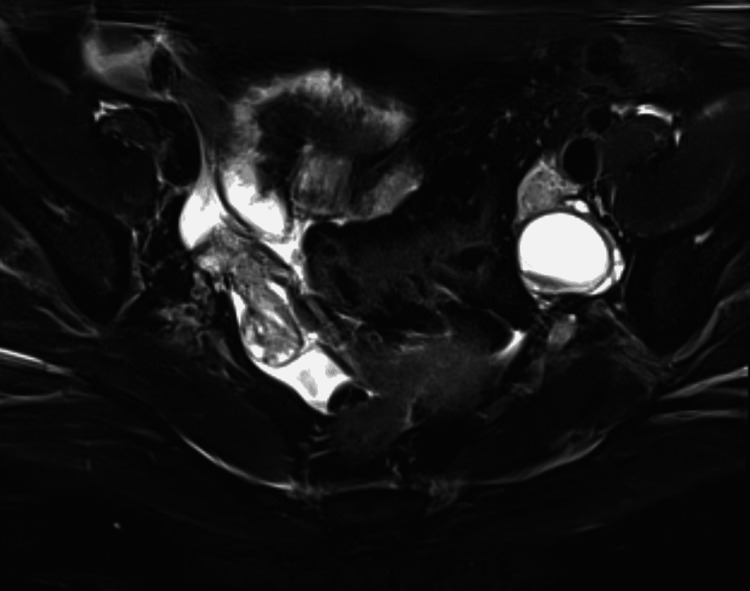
Pelvic MRI showed an unilocular cystic lesion with focal calcification and a small amount of sludge inside the left ovarian cyst. However, restricted diffusion, abnormal enhancement, and heterogeneous composition were not observed.
